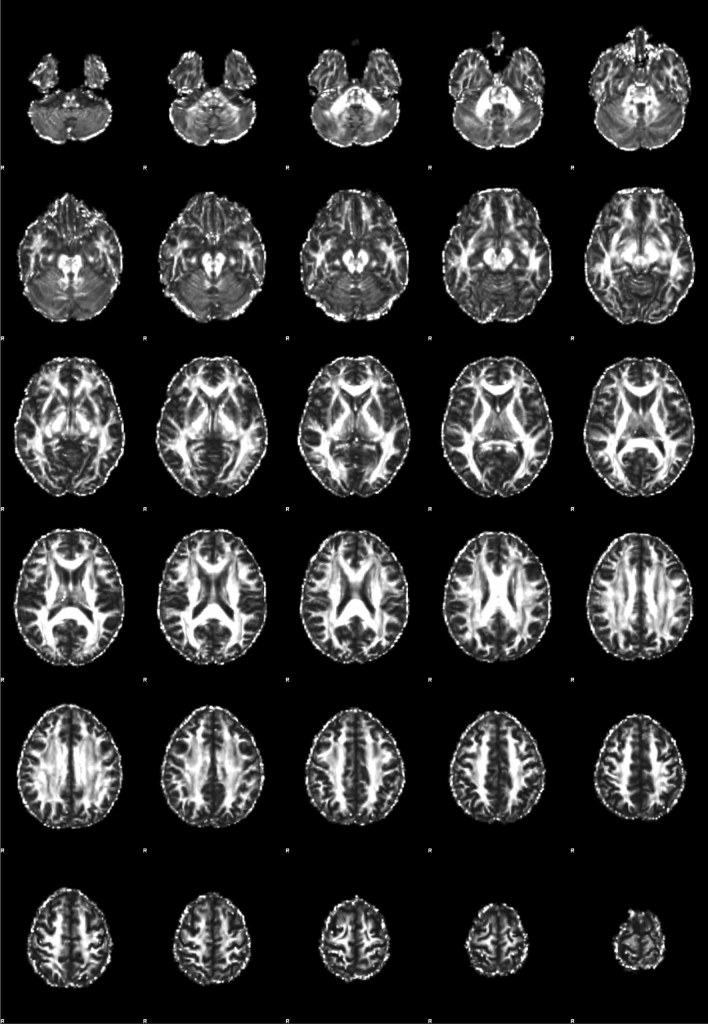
MRI slices of subjects with the largest FA-BHQ.
